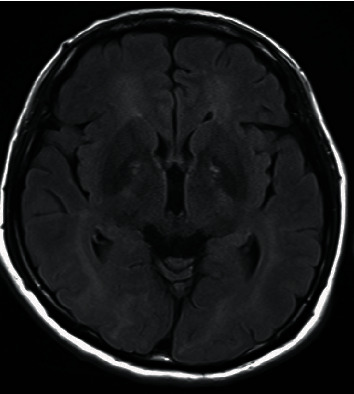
Magnetic resonance imaging (MRI) 40 days after the suicide attempt of case 2.
